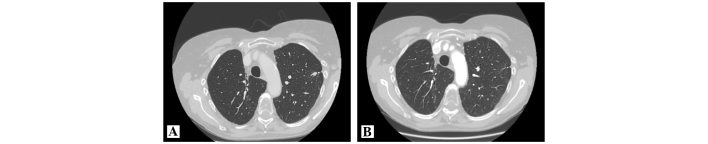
Comparison of (A) the initial computed tomography (CT) of the thorax and (B) the repeat CT three months after initiation of crizotinib therapy, demonstrating the dramatic shrinkage of the tumour lesions.
